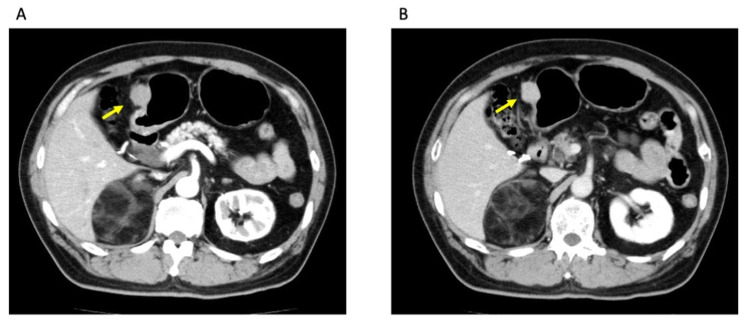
Contrast-enhanced CT (CECT) Contrast-enhanced CT (CECT): A: early phase, B: late phase. CECT shows a solid tumor with mild vascularity in the stomach wall (arrows).
